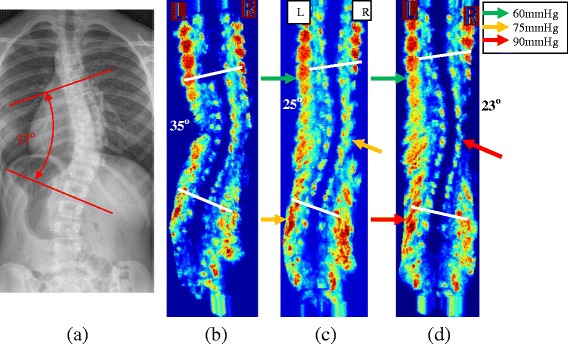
a The standing pre-brace X-ray with Cobb angle 37°. b The baseline US scan (Cobb angle 35°). c The first trial US scan (Cobb angle 25°). d The 2nd trial US scan (Cobb angle 23°)
